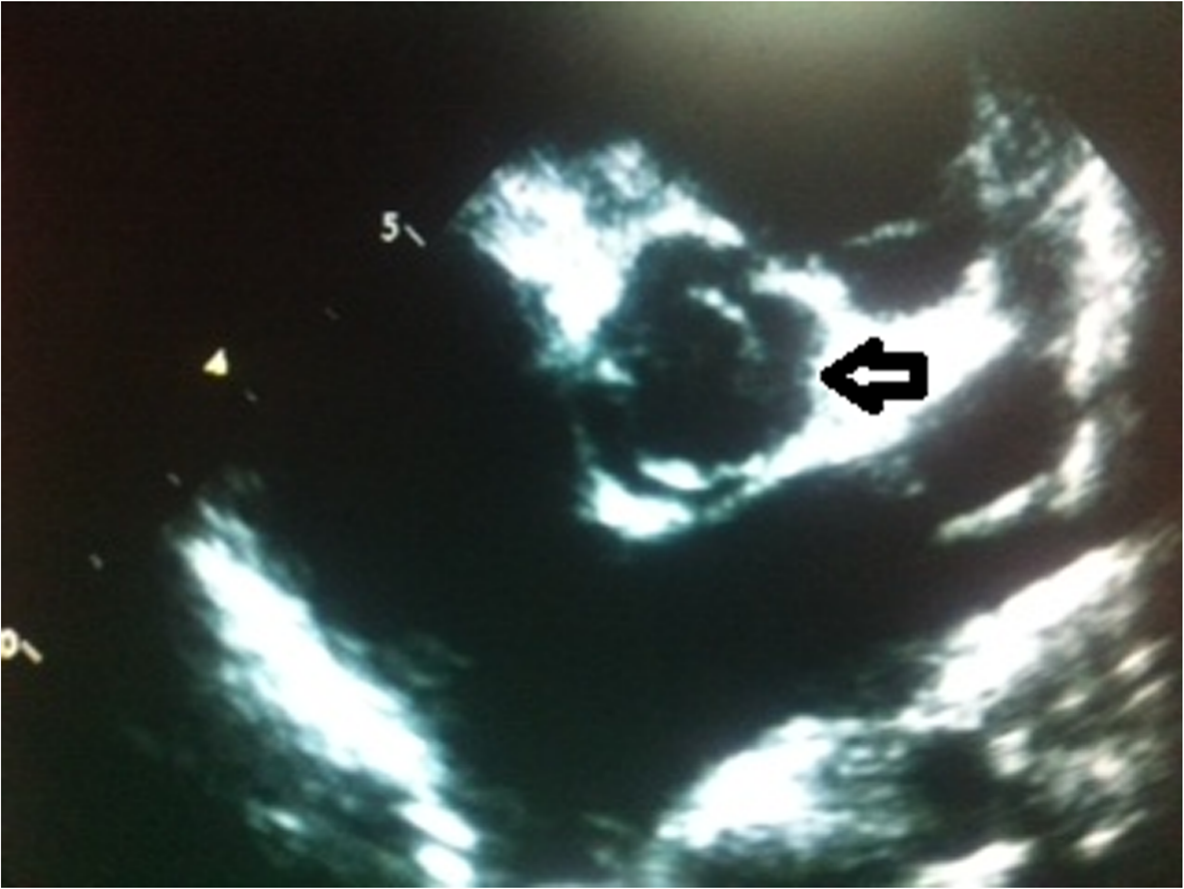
Echocardiography short axis parasternal view showed bicuspid aortic valve.
